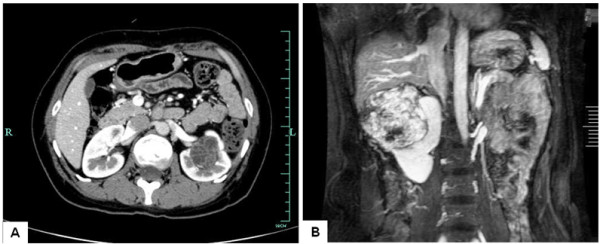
Representative radiological findings of mixed epithelial and stromal tumor of the kidney. (A) Patient 3. Abdominal computed tomography scan showed a left renal tumor with cystic and solid components. (B) Patient 7. T2-weighted coronal magnetic resonance imaging showed a giant, well-circumscribed, multi-cystic tumor that had originated from the right kidney.
